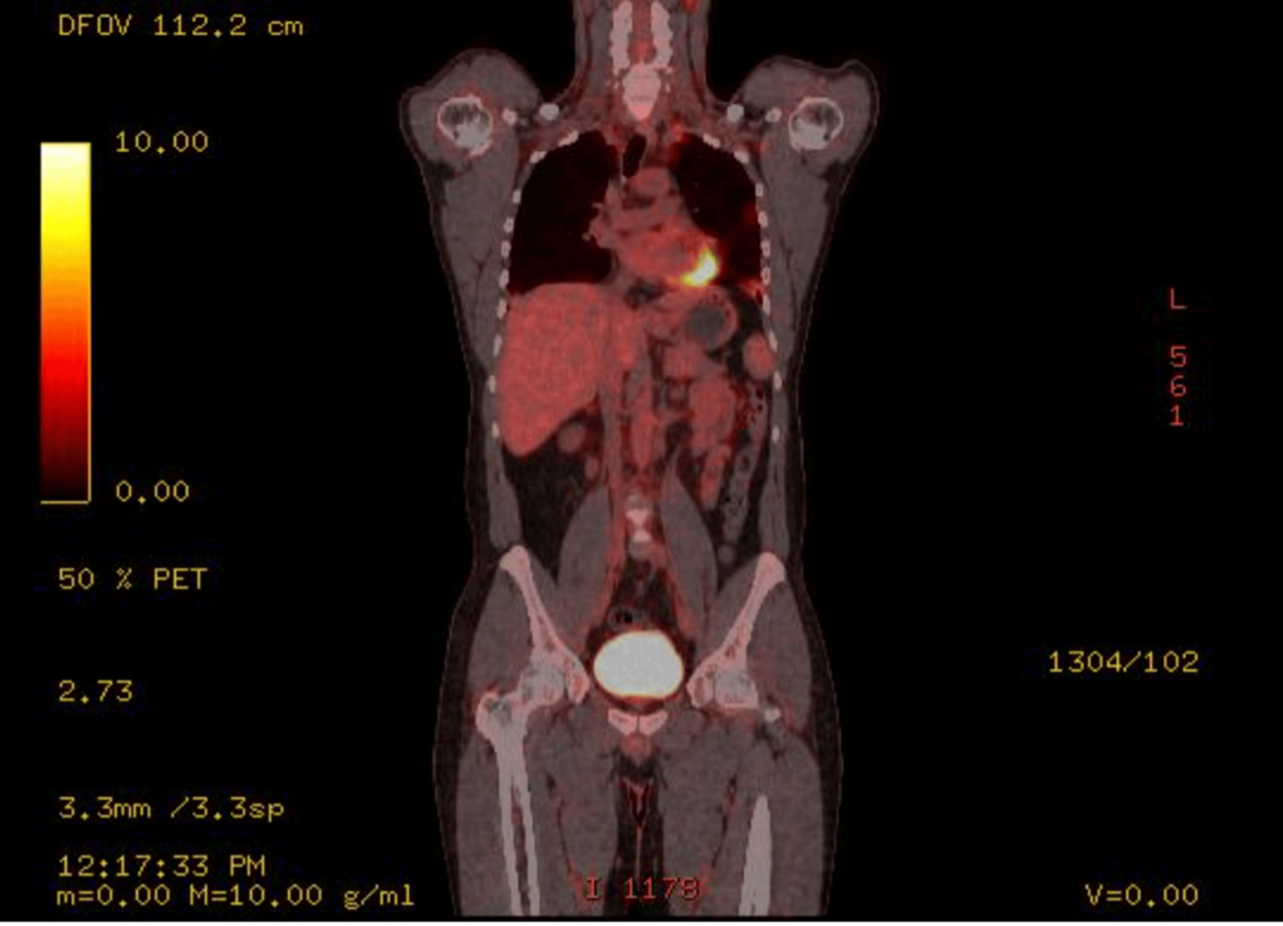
PET-CT post chemotherapy showing complete regression of the disease
